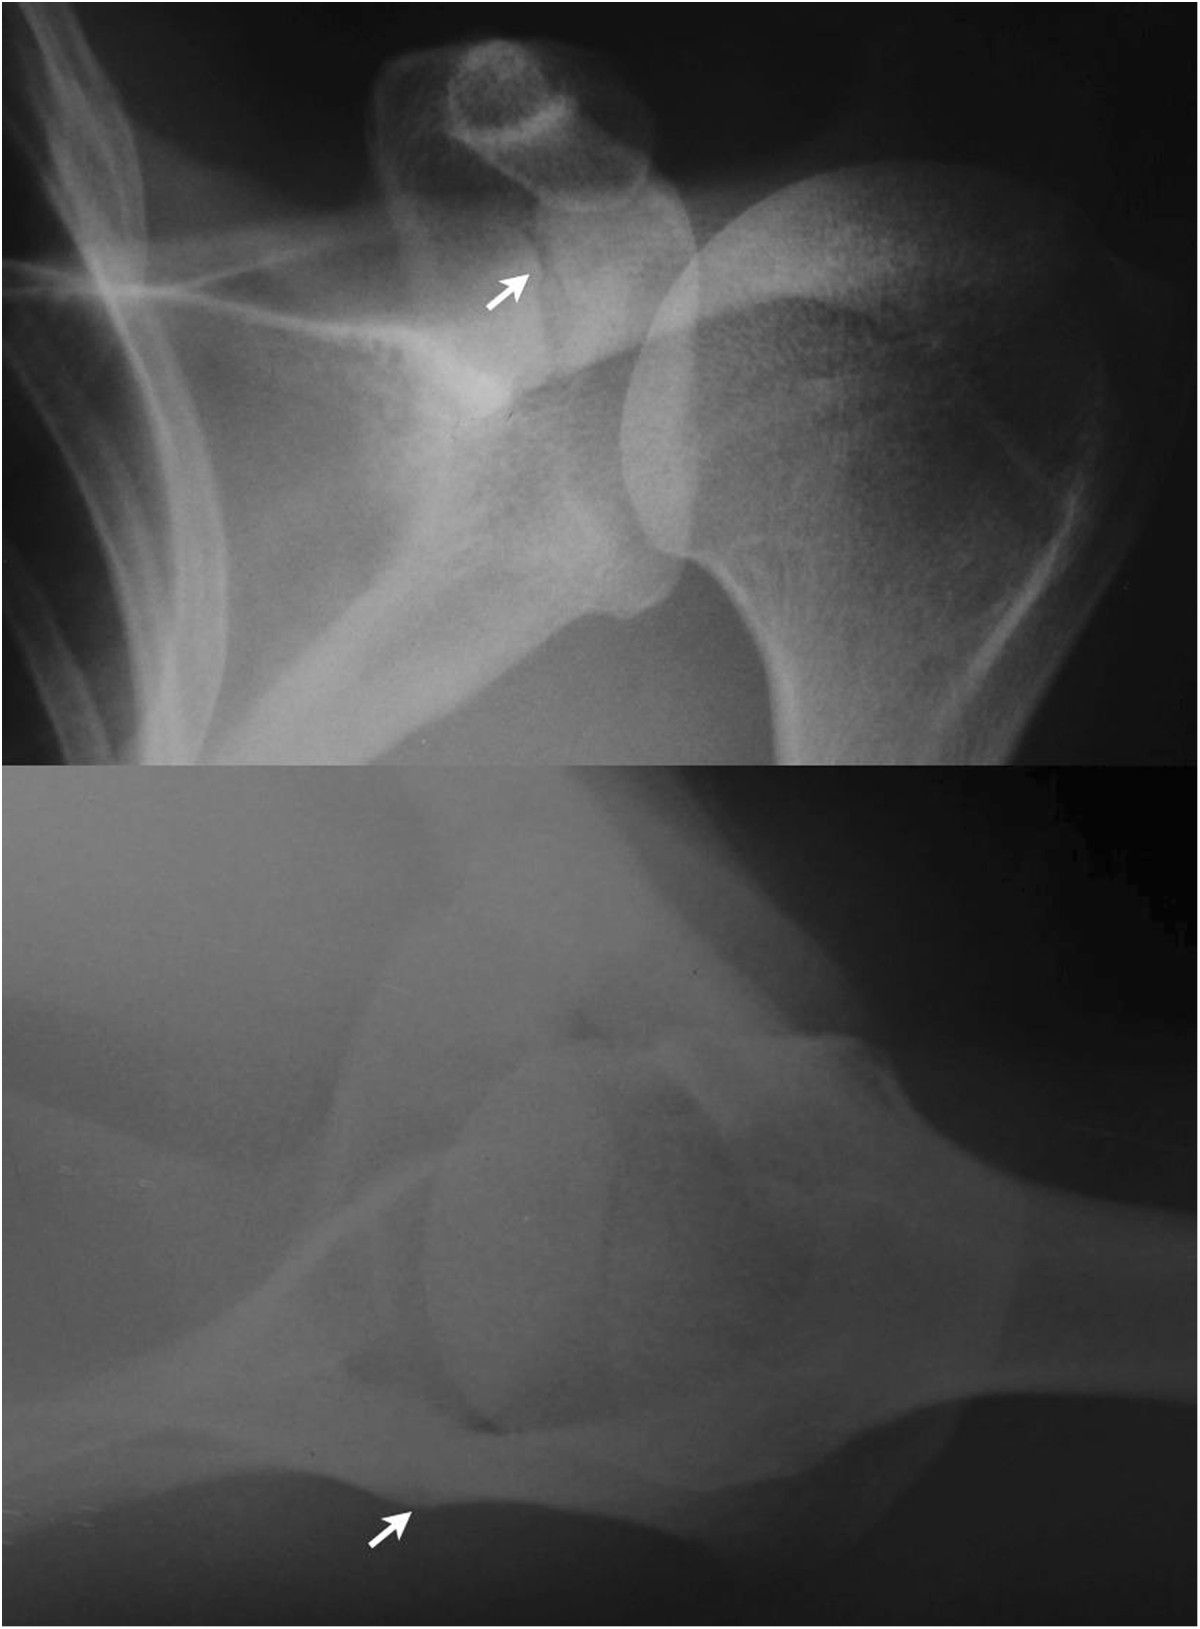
Preoperative radiographs. Radiographs in the anteroposterior and axillary views demonstrating (arrows) the transverse fracture and the lack of deviation of the base of the acromion.
